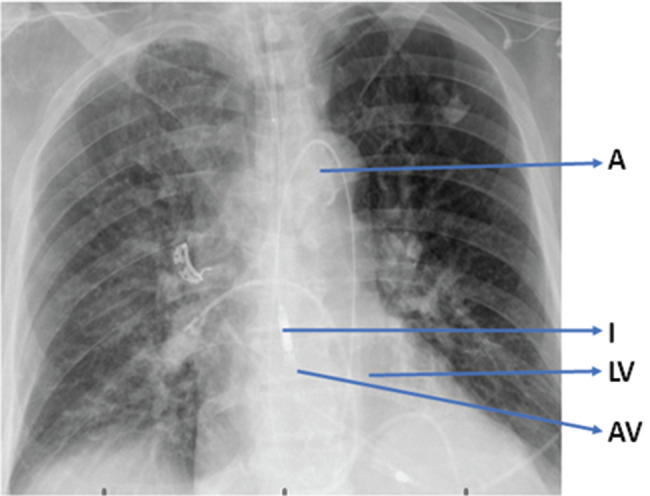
Chest X-ray of the Impella^®^ device lying across the aortic valve into the left ventricle. *Abbreviations:* A, aorta; AV, approximate position of the aortic valve; I, Impella^®^; LV, left ventricle.
